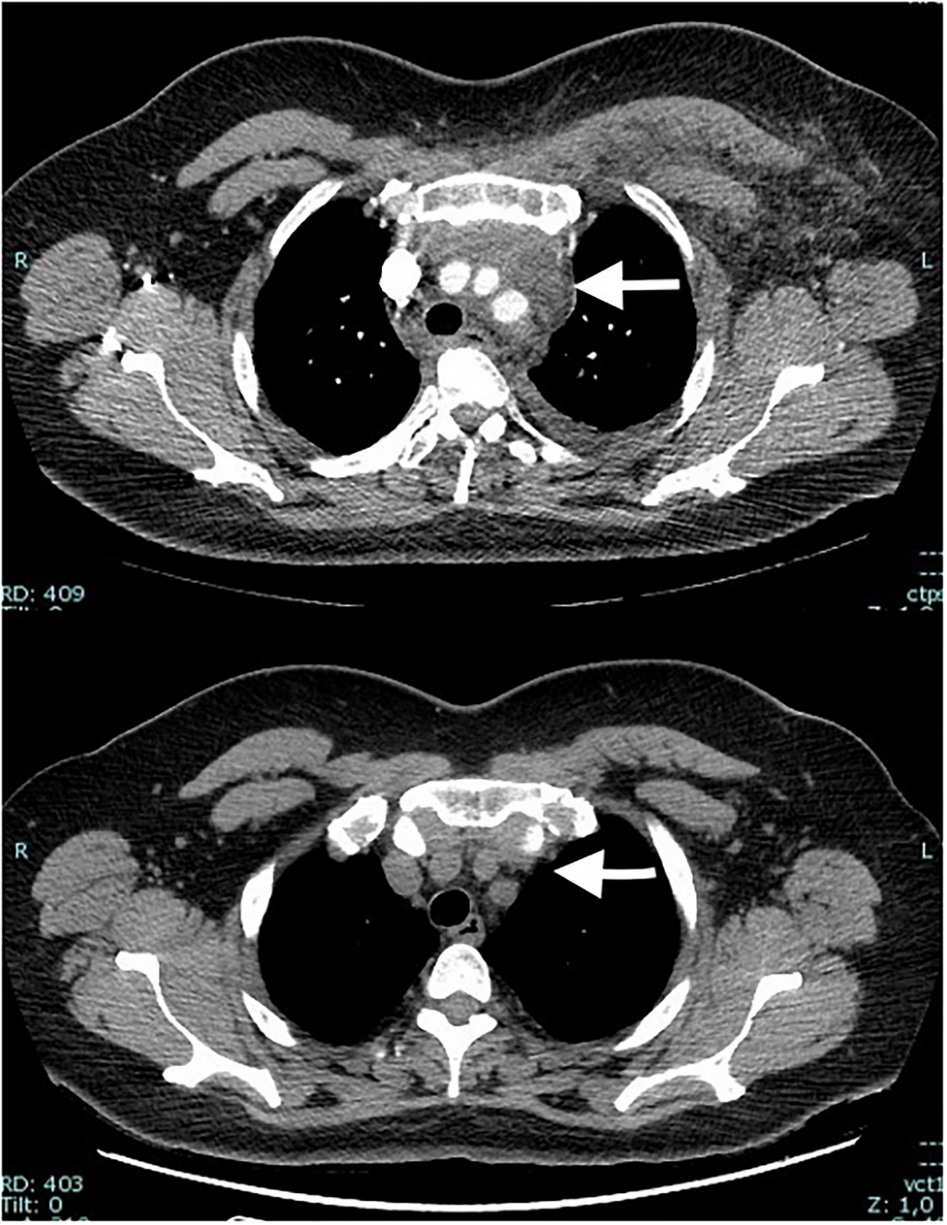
Contrast medium Chest Computed Tomography showing a mass of the upper anterior mediastinum compressing and displacing the left anonymous vein that is occluded (above) (white arrow). After 6 weeks, after the resolution of the SARS-CoV-2 the mass disappeared (white arrow).
